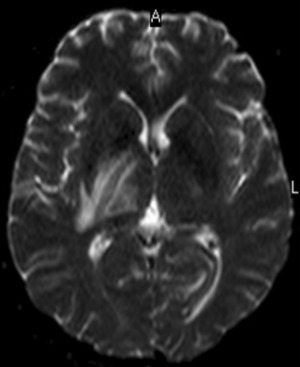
Magnetic resonance image of brain paraventricular area
